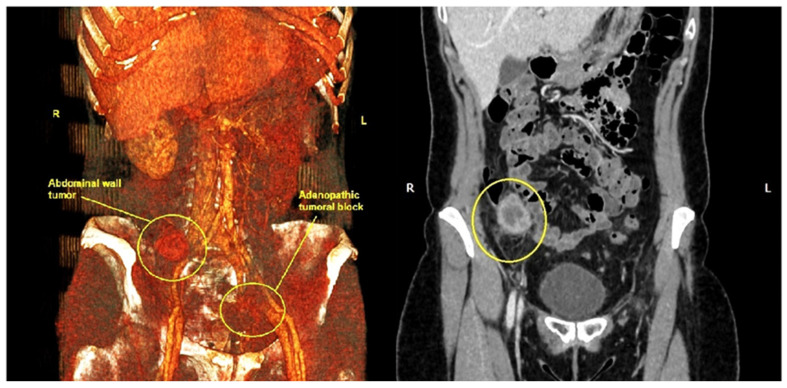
Abdominal CT scan with 3D reconstruction shows the parietal tumor from the right iliac fossa and the left iliac adenopathic block (yellow circles). (R—right, L—Left).
